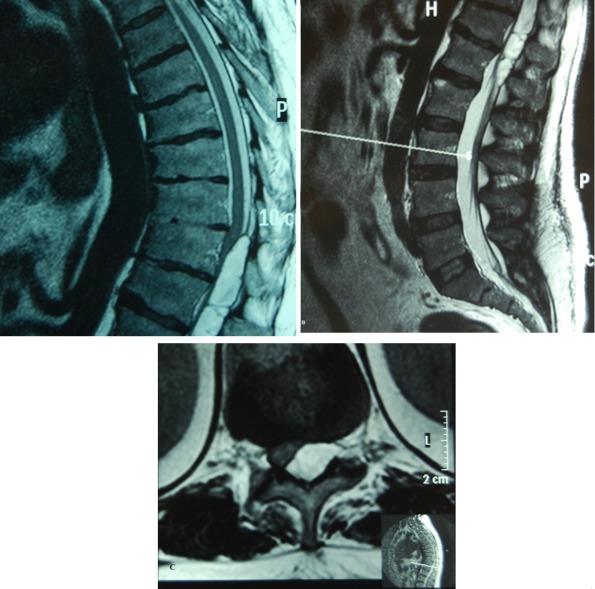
A, B) Sagittal T1W MRI images showing a well defined lesion in the posterior epidural space extending from T10 to L2 level, C) Axial T1W MRI image showing a hyperintense lesion in the posterior epidural space displacing the cord anteriorly and compressing it.
